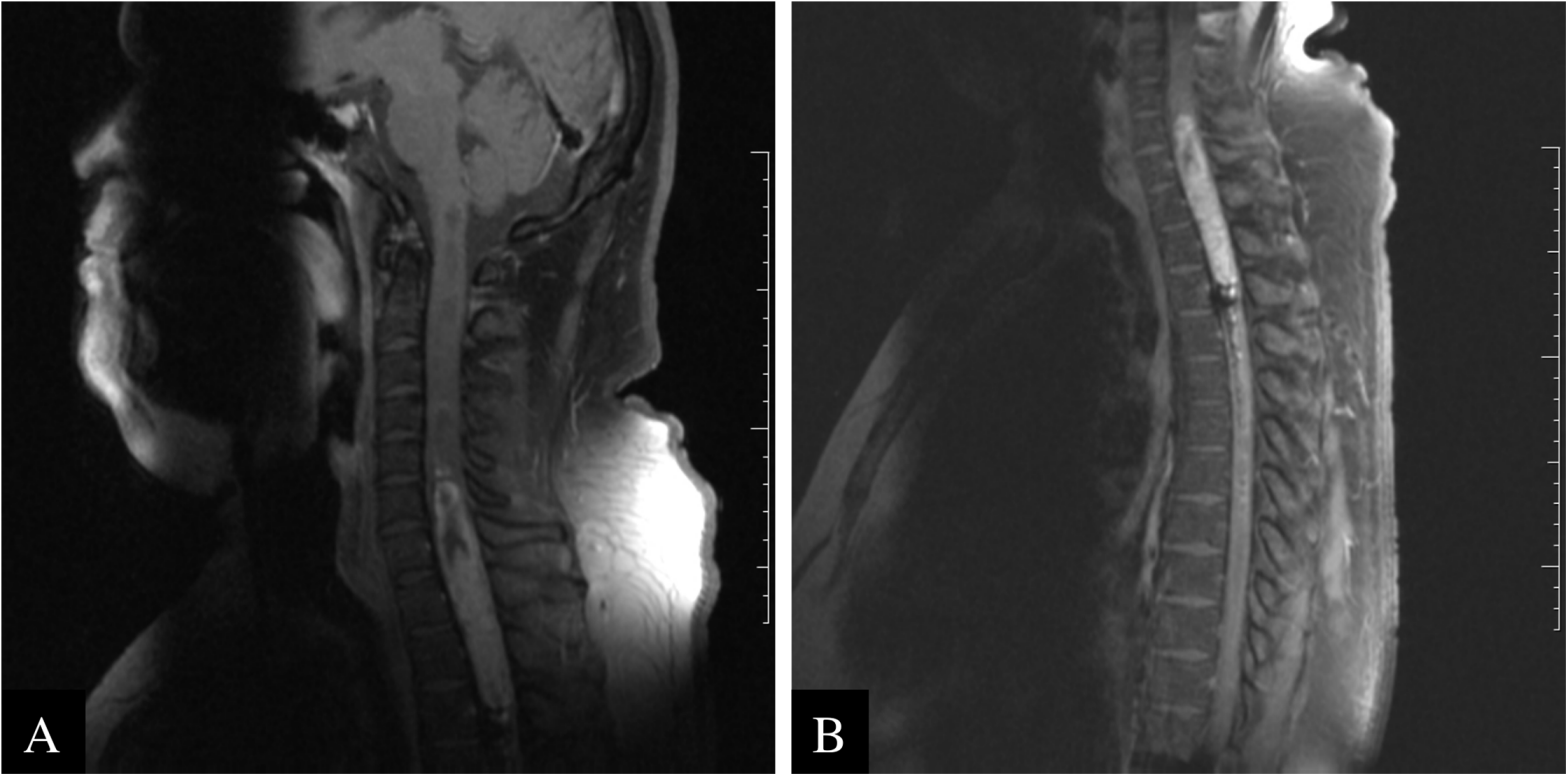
Imaging features of the RGNT. (A and B) Magnetic resonance images showing an expansive lesion between C6 and T3.
